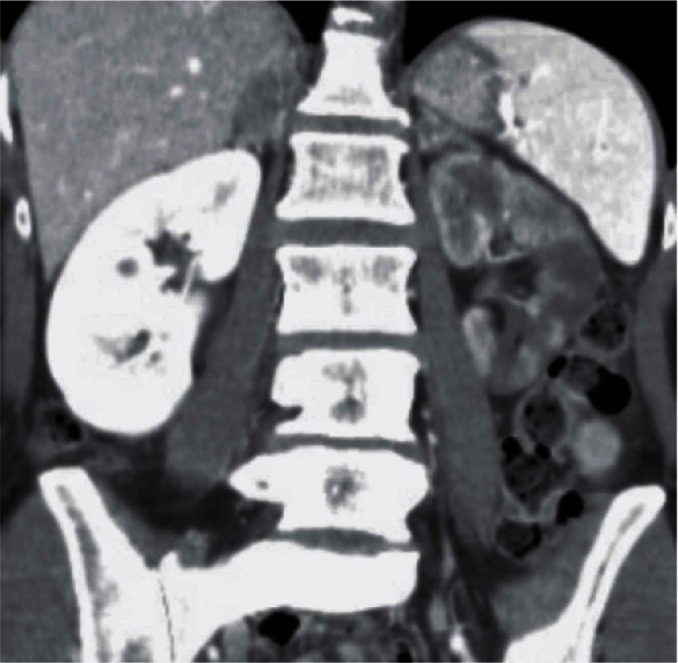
CT angiogram showing normal findings on the right side but the left kidney is severely hypoperfused with probably multiple renal infarcts.
